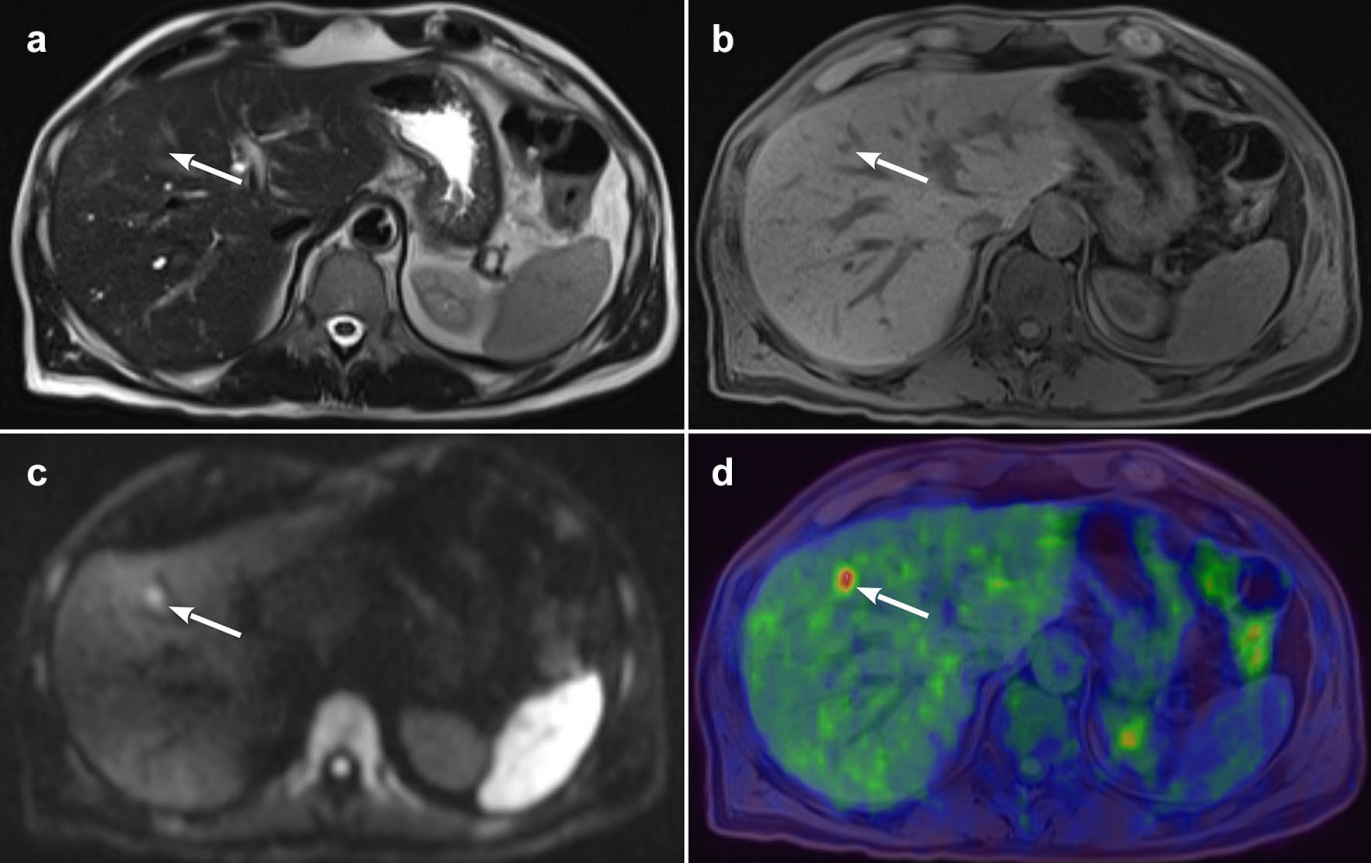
PET/MR images of a small liver metastasis. a
